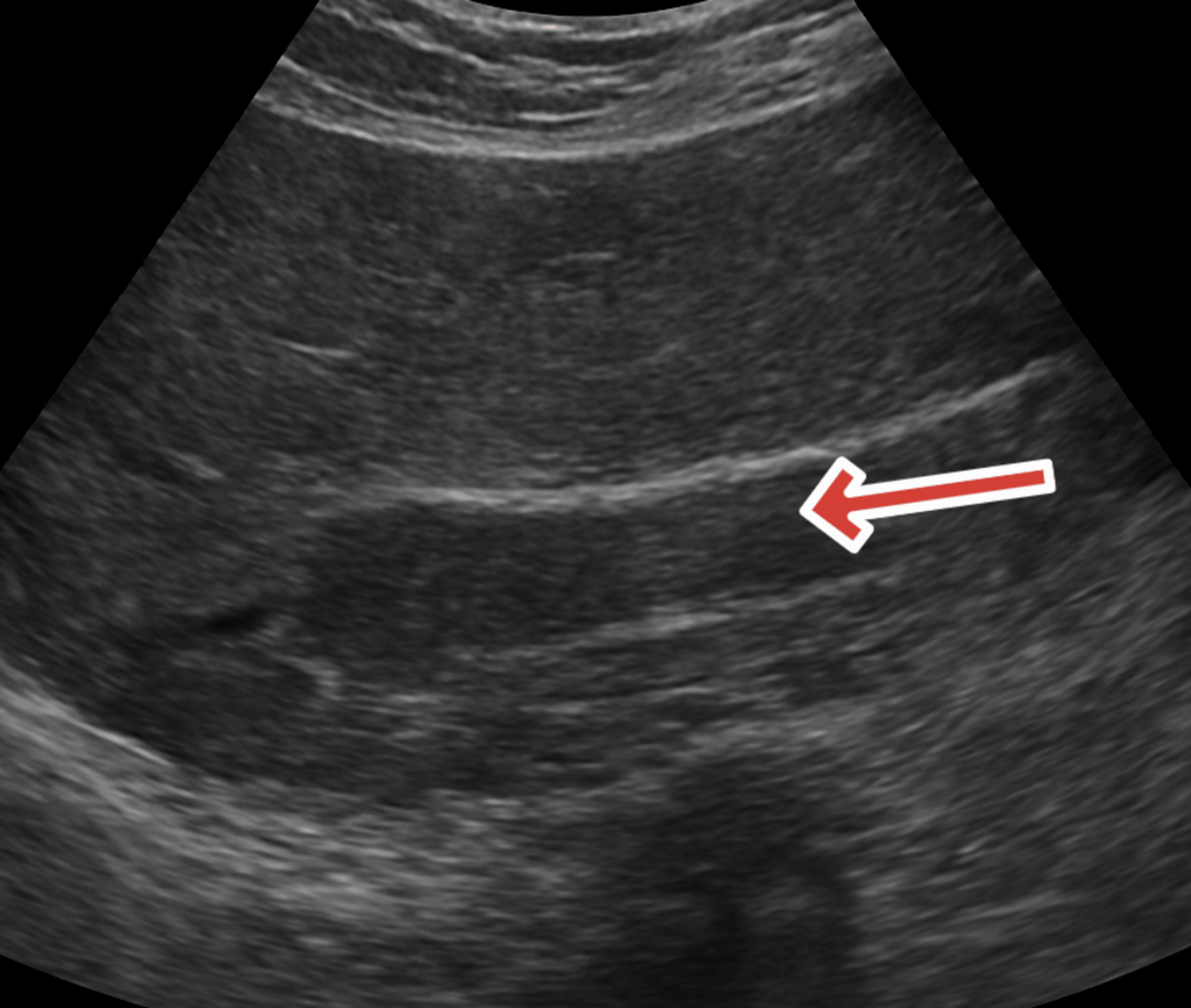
Abdomen ultrasound showing convalescent phase pancreatitis (Jan 02, 2025) The red arrow shows the subsiding and resolving phase of pancreatic tissue.
